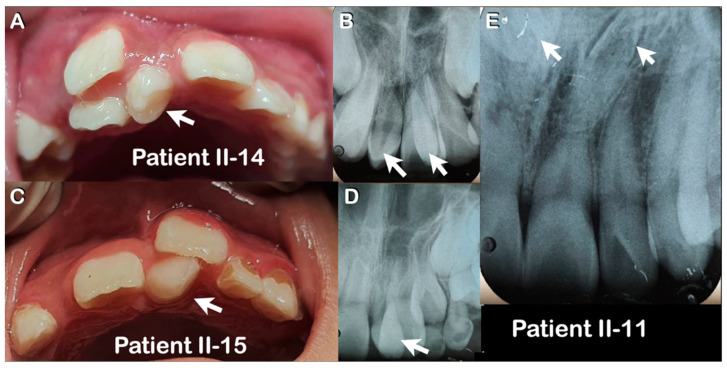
Clinical and radiographic pictures of mesiodens (arrows) in (A) Patient II-14. (B) Periapical radiograph in (A) shows double mesiodentes (arrows). (C) Patient II-15. (D) Periapical radiograph in (C) shows mesiodens. (E) Periapical radiograph of patient II-11. Note double inverted mesiodentes (arrows).
